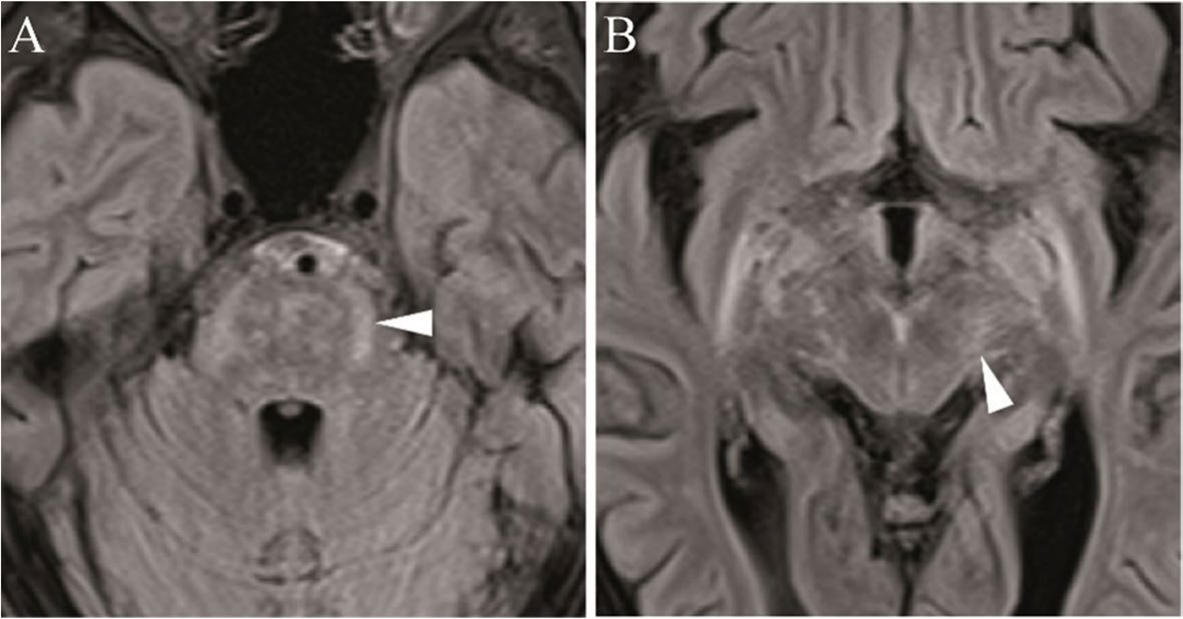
Magnetic resonance imaging (MRI) of the brain of Case A-B: Axial slices with fluid-attenuated inversion recovery (FLAIR), showing brainstem lesions spreading into the midbrain with an inflammatory appearance
